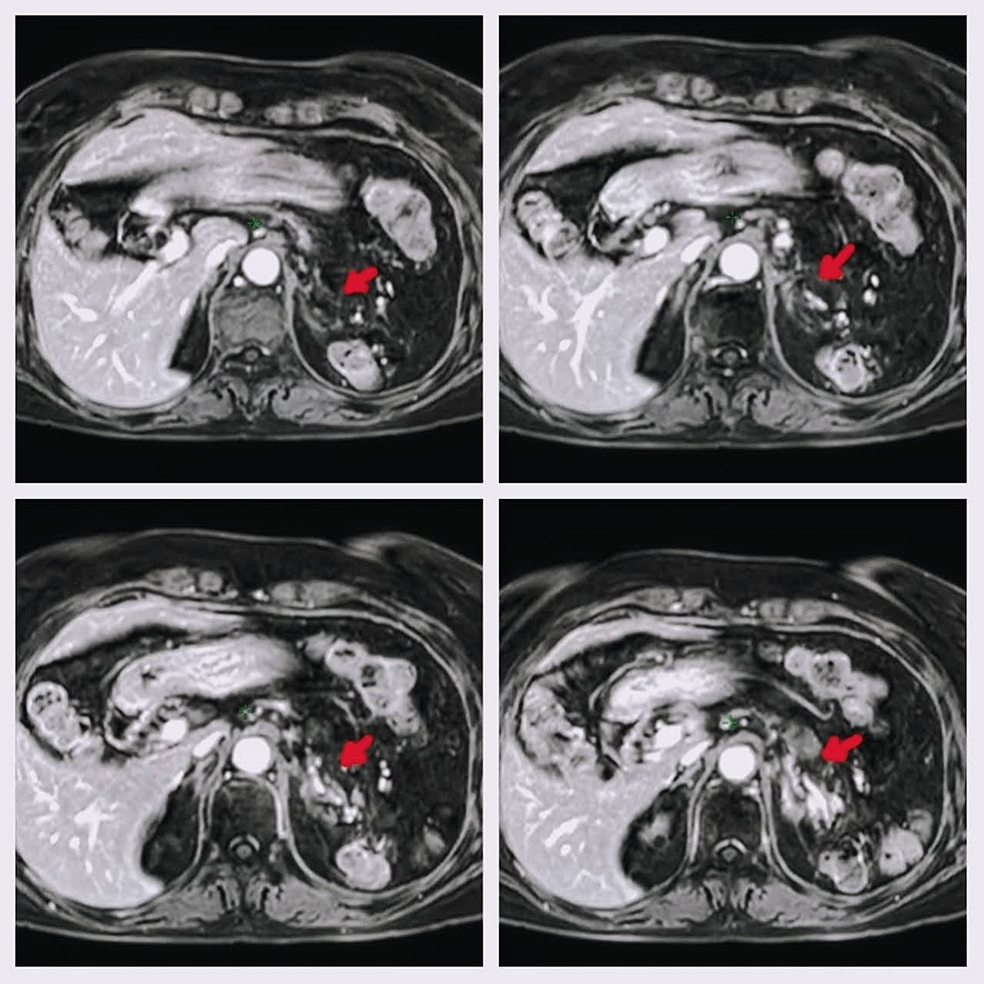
Post-treatment evaluation abdominal MRI The images show hypointense bed (arrow) that has remained after the treatment in the areas where they were previously occupied by the hyperintense mass. Hyperintense remnants can also be identified, especially near the vertebral body. However, a significant decrease in the size of metastasis can be identified.
